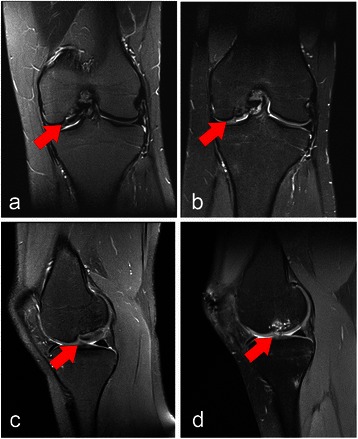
Preoperative and postoperative magnetic resonance imaging of the patient’s left knee. Coronal (a and b) and sagittal (c and d) t2-weighted magnetic resonance imaging images of her left knee preoperative with osteochondral lesion (see arrows) and 12-month postoperative with refixation of the lesion showing the repaired tissue (see arrows)
